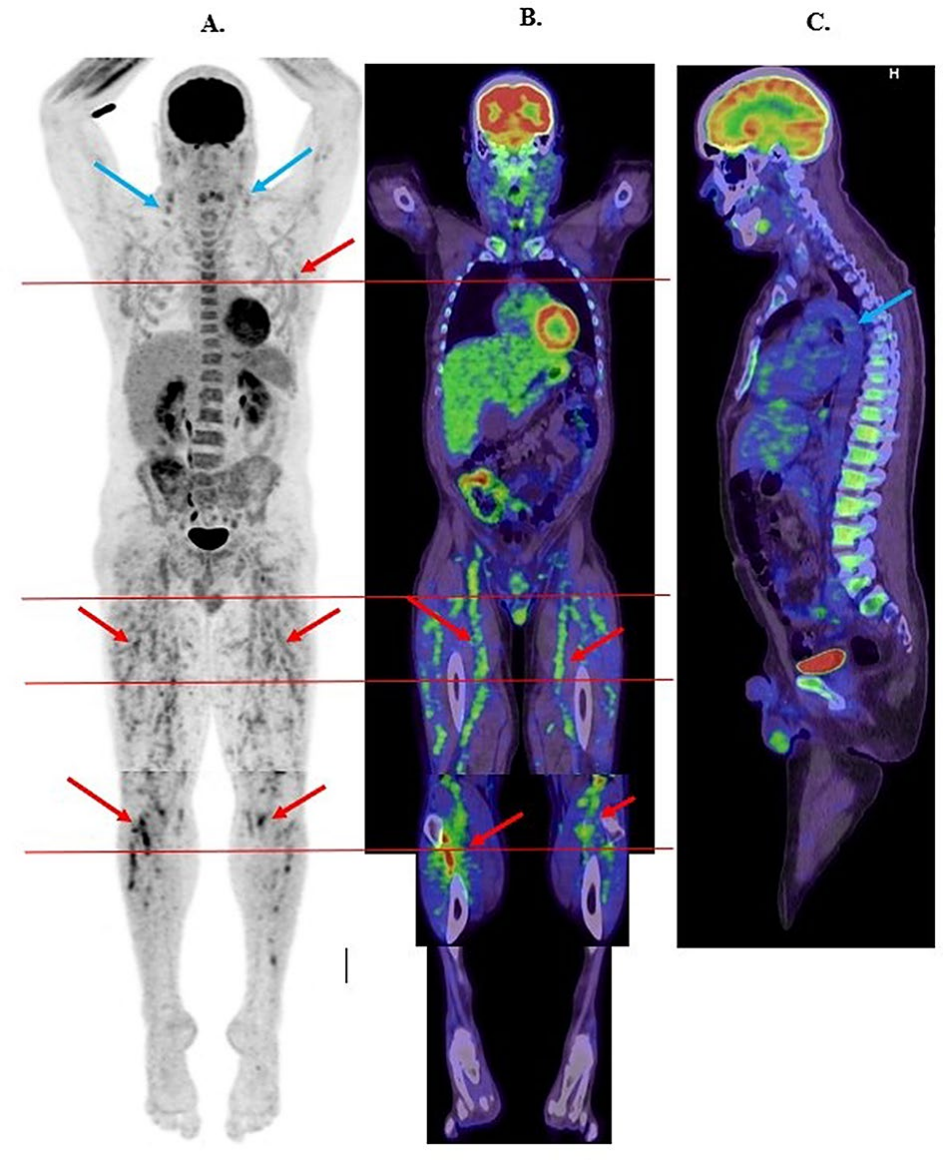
A Whole body ^18^F-FDG PET Maximal Intensity Projection image demonstrating widespread medium vessel vasculitis predominantly involving both lower limbs (thighs and proximal calves) and much lesser proximal upper limbs / axillae (red arrows). Moderate FDG uptake in bilateral cervical nodes also demonstrated (blue arrows). Horizontal red lines depict different trans-axial slice levels demonstrated in Fig. 2. B Whole body ^18^F-FDG PET/CT coronal fused images demonstrating medium vessel vasculitis involving bilateral femoral and popliteal vessels and numerous smaller branches throughout the thighs and proximal calves (red arrows). Horizontal red lines depict different trans-axial slice levels demonstrated in Fig. 2. C Whole body ^18^F-FDG PET/CT sagittal fused images demonstrating no abnormal FDG uptake in the ascending or descending thoracic aorta (blue arrow)
